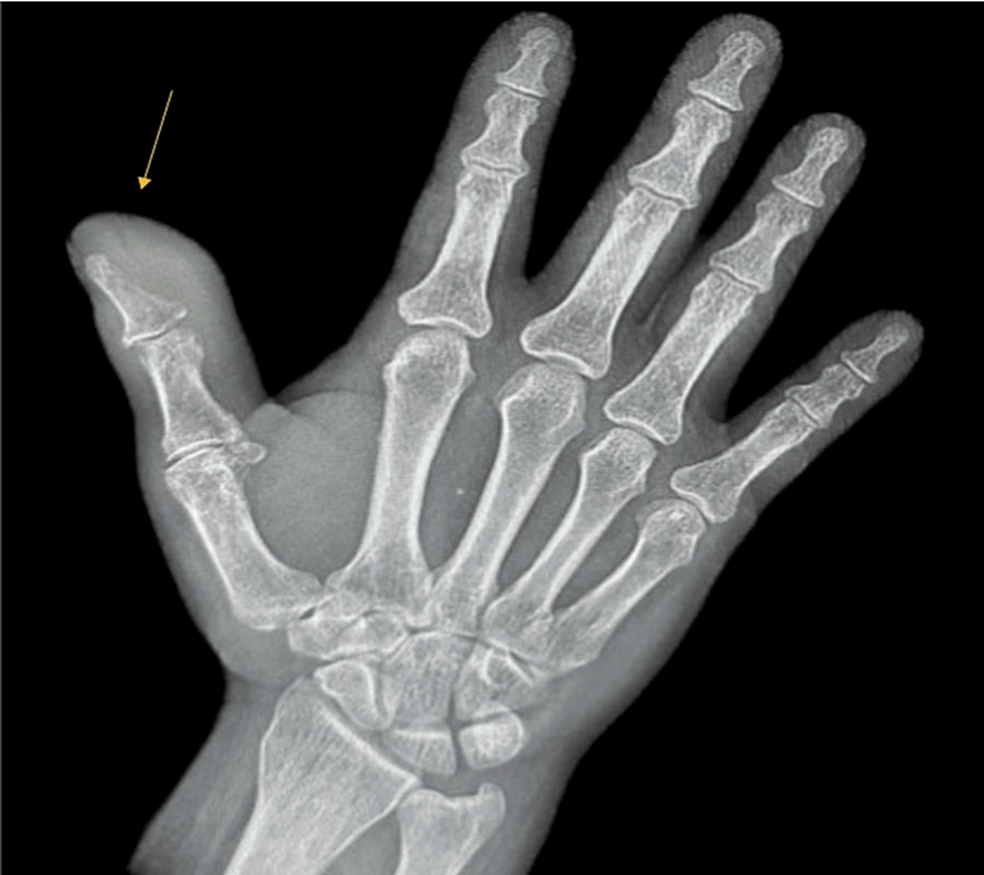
X-ray of the right hand showing soft tissue swelling involving the first digit.
